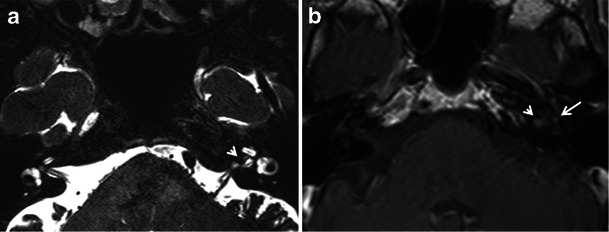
a Axial CISS MR image of the skull base in an adult patient with left-sided SNHL. A small hypointense filling defect is seen in the left internal auditory canal (*arrowhead*), which may be suggestive of an acoustic neuroma. b Axial contrast-enhanced MR image of the same patient as (a), at the same level. The previously noted filling defect in the left internal auditory canal shows post-contrast enhancement, which indicates a small acoustic neuroma (*arrowhead*). There is also a suggestion of enhancement in the left pericochlear region and in the region of the left fissula ante fenestram (*arrow*), which suggests associated otosclerosis is present
